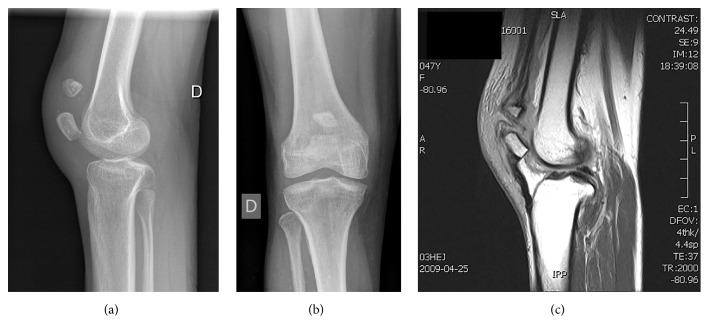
Lateral (a) and anteroposterior (b) knee views demonstrating a gap between the two parts of a duplicate patella. (c) Soft tissue is identified between the two parts of the patella (MRI T1 sagittal view).
